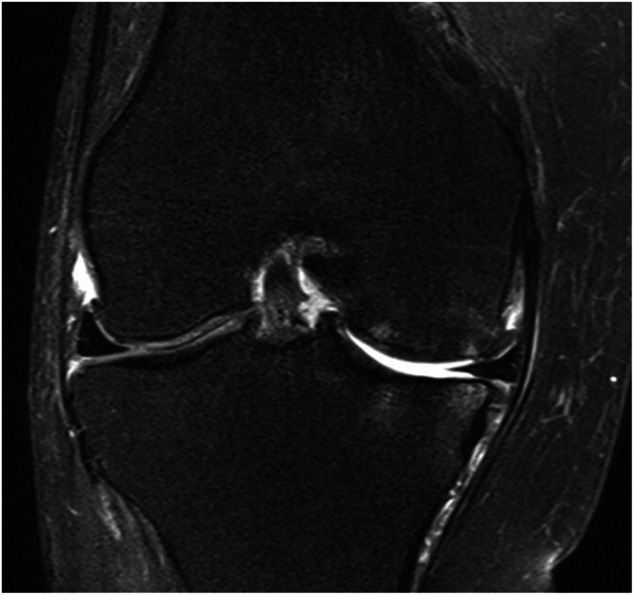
Coronal MRI of the knee of natural history patient #3 showing progression of osteoarthritis over 5 months following neglected medial meniscal root tear (right knee).
